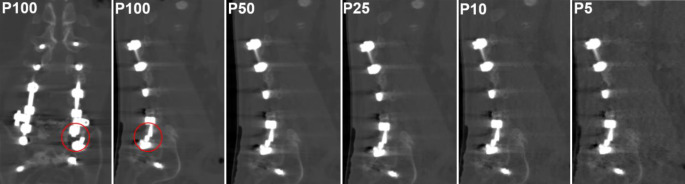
Multidetector computed tomography (MDCT) images of a patient with implant failure after dorsal stabilization using a rod-screw system (spanning L1–S2). Coronal and sagittal MDCT reconstructions with statistical iterative reconstruction (SIR) are shown using 100% of projections (P100), and using sparse sampling with 50% (P50), 25% (P25), 10% (P10), and 5% (P5) of original projections. There is a left-sided rod defect (*red circles*) at the S1 level that is clearly depicted up to a dose reduction of at least 75% (P25)
